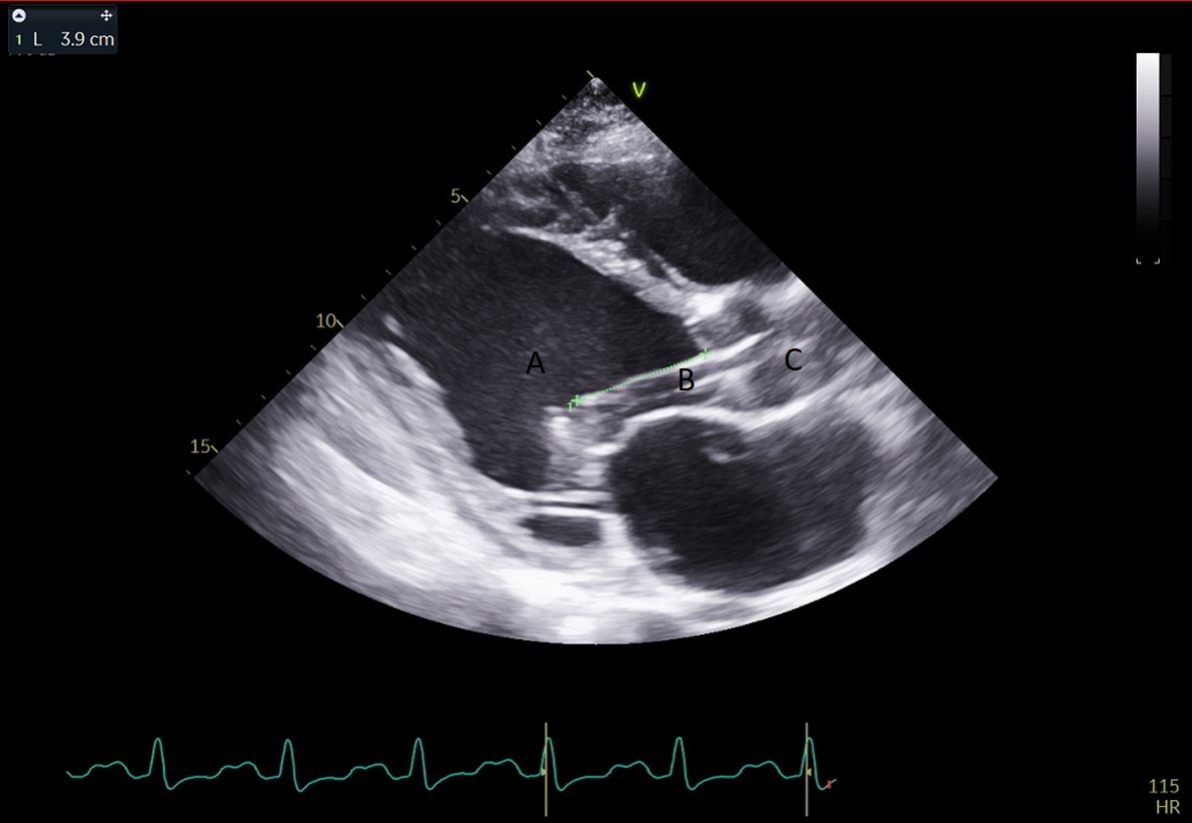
A parasternal long axis view on a transthoracic echocardiogram. The distance from the Impella inlet to the aortic valve is measured and noted to
